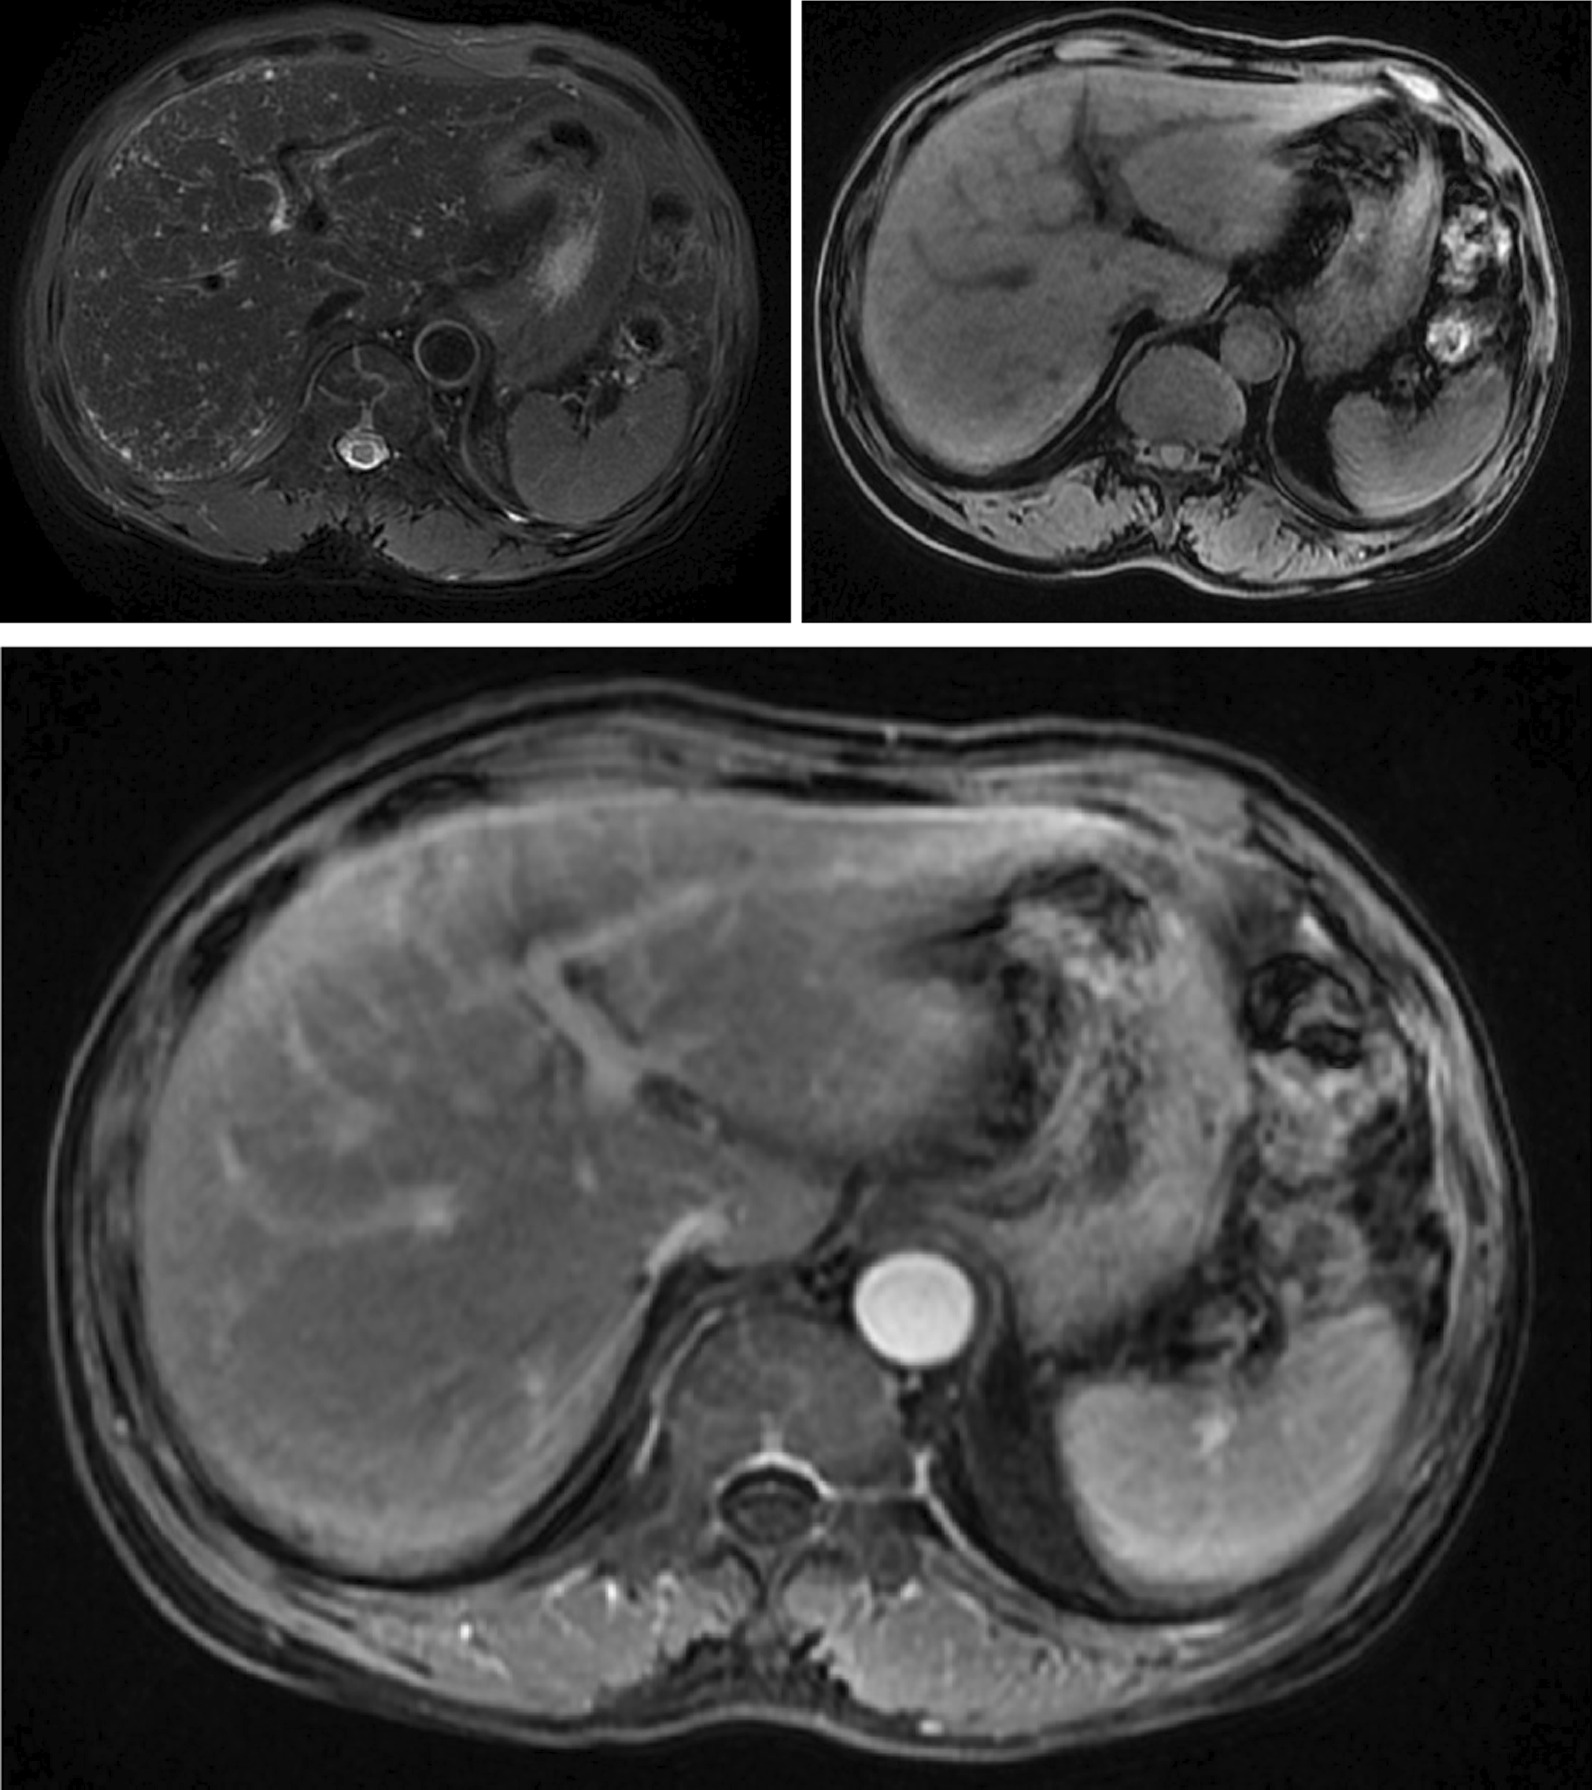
Enhanced magnetic resonance imaging of the liver. The liver images before and after contrast injection showed diffuse intrahepatic lesions
